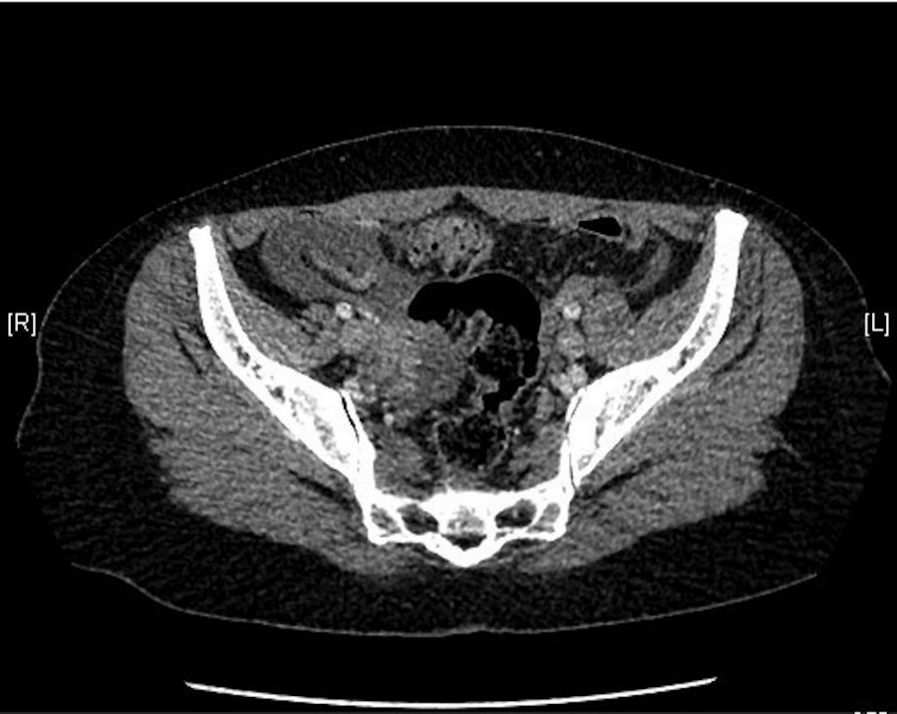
Abdominal CT image showing free pelvic fluid
